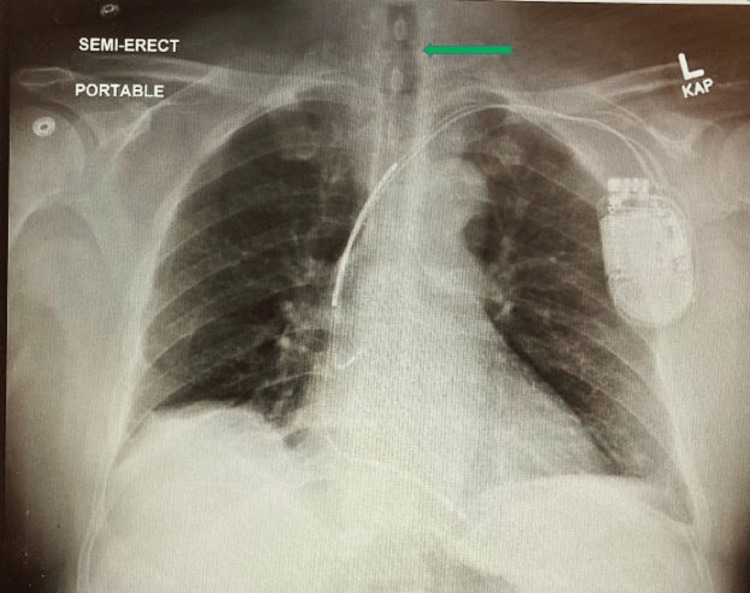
A chest X-ray done in the PACU shows subglottic stenosis with a decreased and irregular air column shown by a green arrow. PACU: Post-anesthesia care unit
